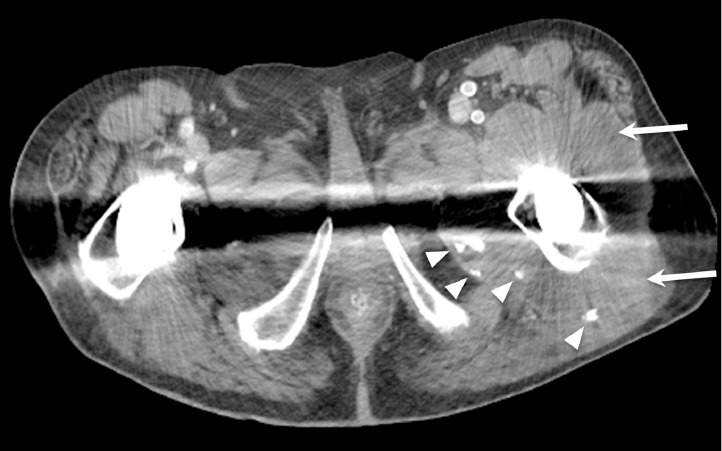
Axial CT image of the pelvis demonstrates pseudotumor formation (arrows) and multiple foci of hyperdense metallic debris (arrowheads) surrounding the left hip.
